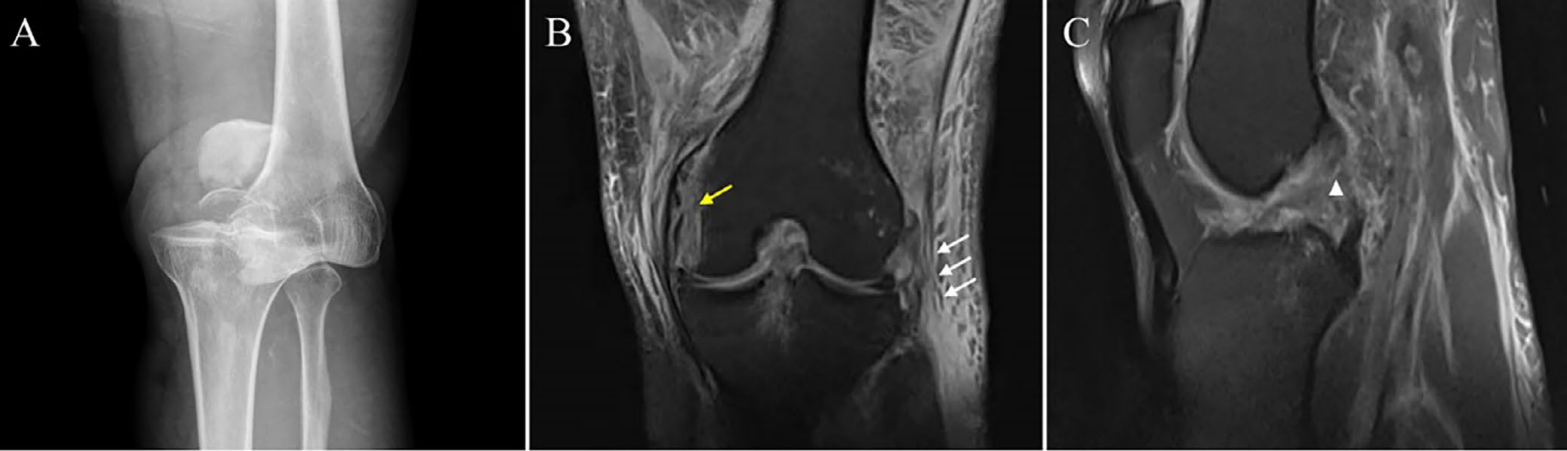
Preoperative imaging results of the patient. (A) Plain radiographs showed the affected knee joint before the reduction of left knee dislocation. (B) MRI indicated injuries to both the lateral collateral ligament (white arrow) and the medial collateral ligament (yellow arrow). (C) MRI showed the disruption of the continuity of the ACL and PCL (white triangle).
